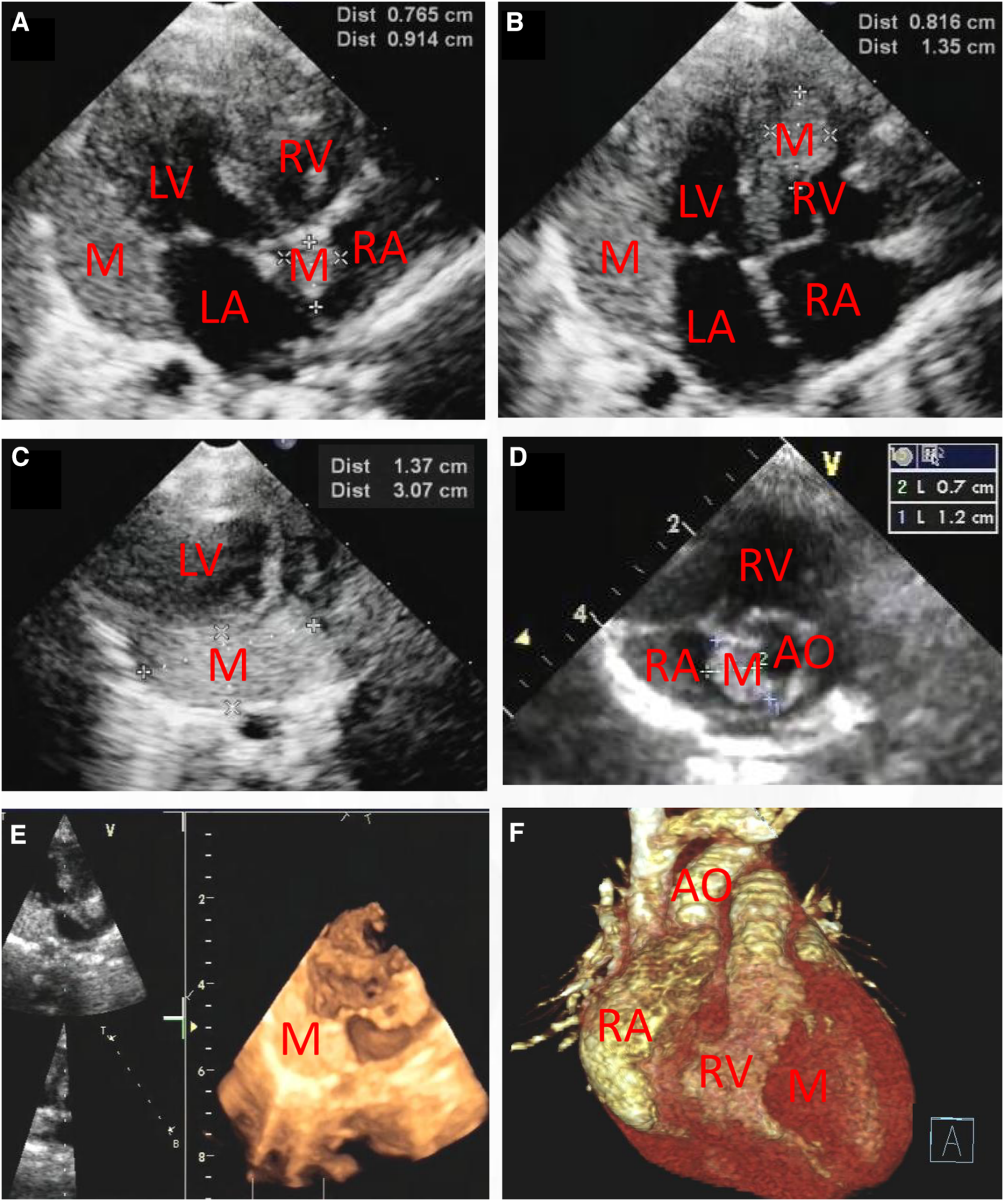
Transthoracic echocardiography (A–D) showing that there are multiple hyperechogenic masses within the atrial septum (0.765 cm × 0.914 cm and 0.7 cm × 1.2 cm), the interventricular septum (0.816 cm × 1.35 cm) and the inferolateral wall of the left ventricle (1.37 cm × 3.07 cm). Three-dimensional echocardiography (E) and Three-dimensional cardiac computed tomography angiography (F) confirmed the detection of cardiac multiple masses. M, mass; RA, right atrium; RV, right ventricle; LA, left atrium; LV, left ventricle; AO, ascending aorta.
